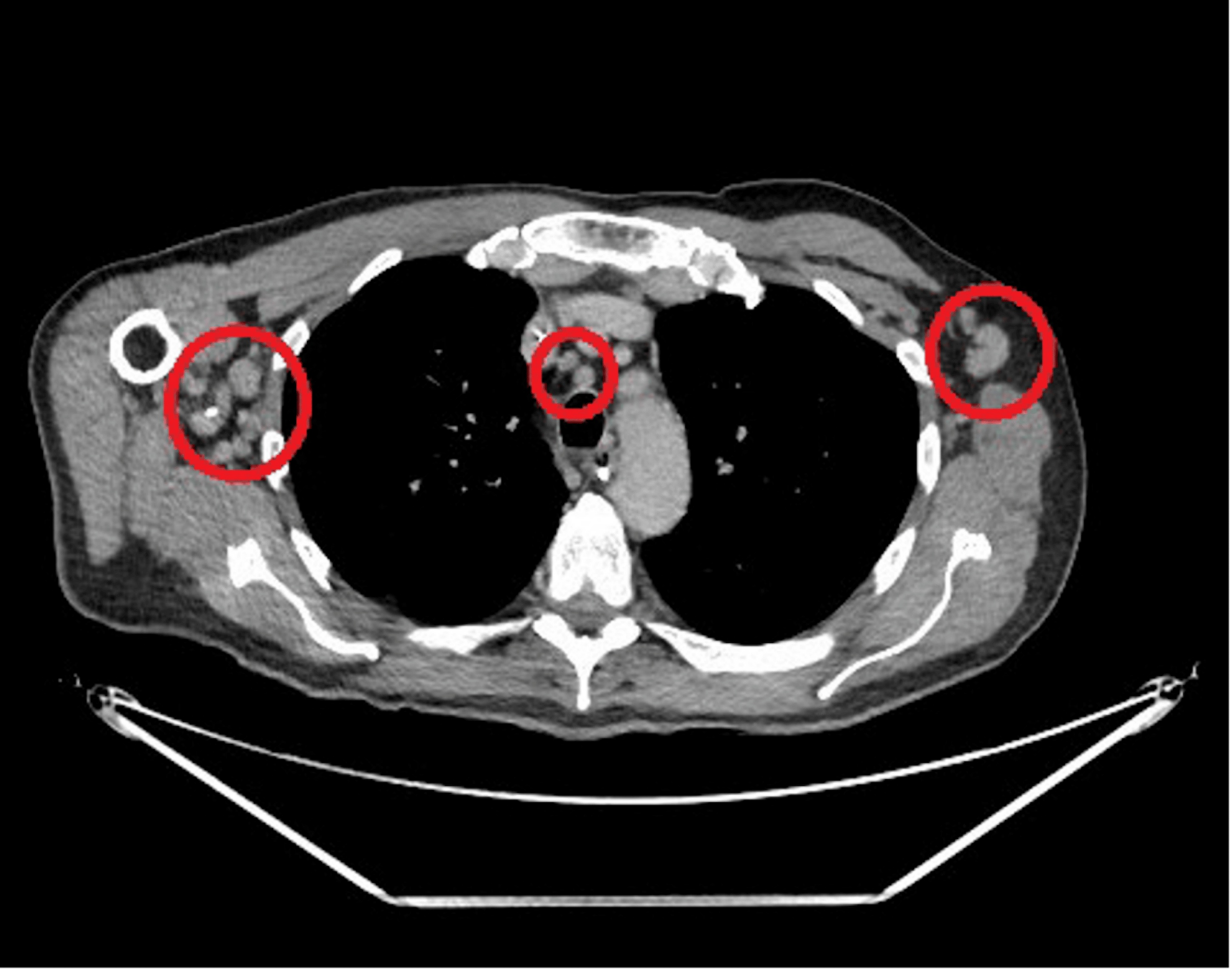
Axillary and pre-tracheal lymphadenopathy indicated on CT-scan The largest nodes seen are 10 mm in diameter CT: computed tomography
